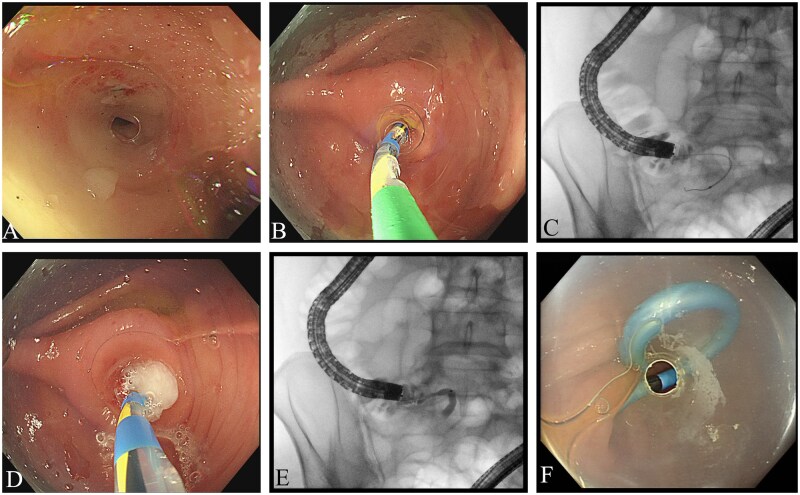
The procedure of endoscopic retrograde appendicitis therapy. (A) Identification of the appendiceal orifice. (B) and (C) Appendiceal intubation using guidewire and catheter techniques under X-ray guidance. (D) Irrigation of the appendiceal lumen. (E) Appendiceal contrast imaging. (F) Appendiceal stent placement.
